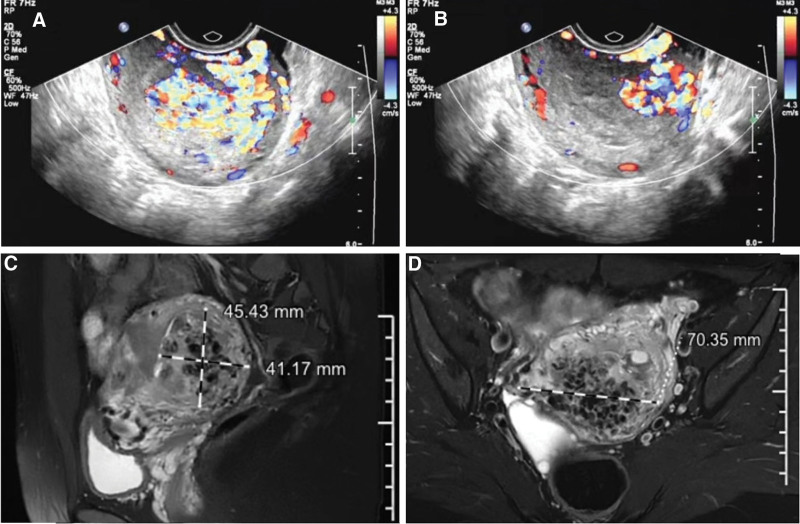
Pelvic TVS (A, B) and MRI (C, D) show a UAVF of the right posterior wall measuring 7.1 × 4.1 × 4.5 cm (case 2). MRI = magnetic resonance imaging, TVS = transvaginal ultrasonography, UAVF = uterine arteriovenous fistula.
